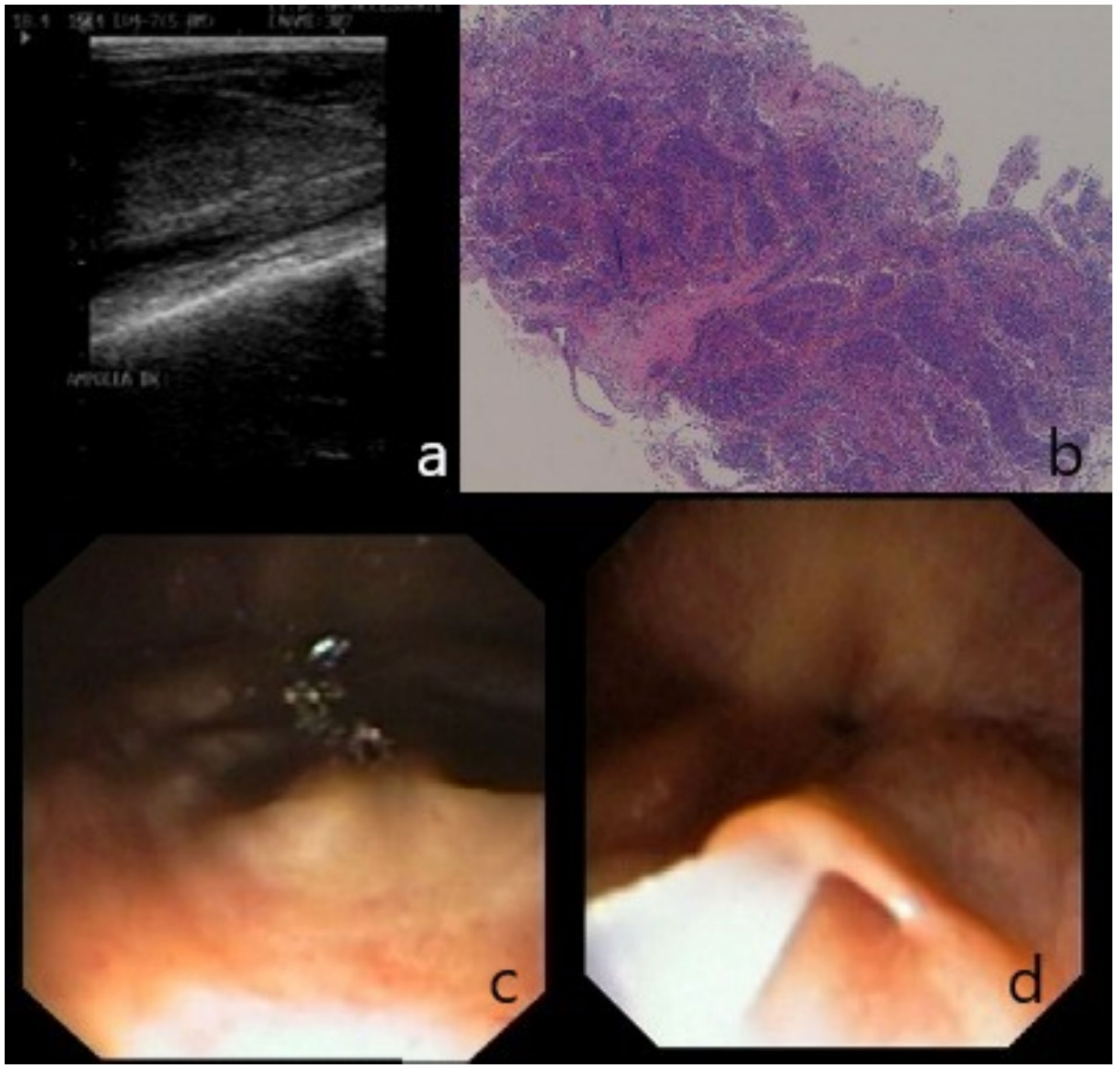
Findings of clinical examination: (a) ultrasonographic visualization of right ampulla; (b) testicular biopsy, spermatogenesis is evident in seminiferous tubules; (c) endoscopic visualization of the urethral opening of ampullae. (d) Detail of ampullae cannulation and insufflation.
